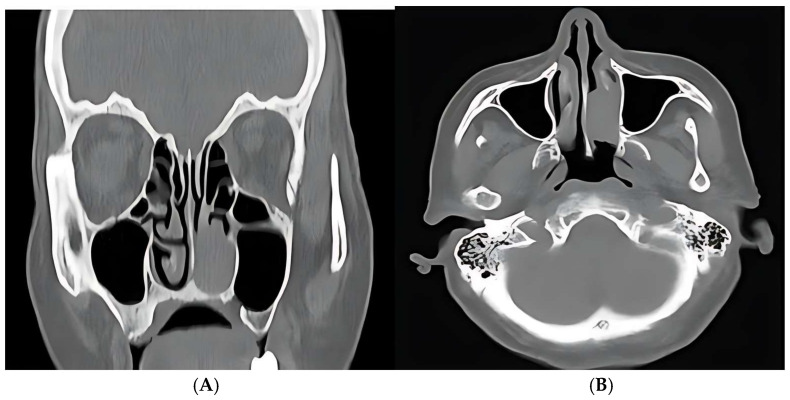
Paranasal CT scan images, coronal (A) and axial (B) view, showing a soft tissue mass in the left nasal cavity, with no bone invasion or local extension.
